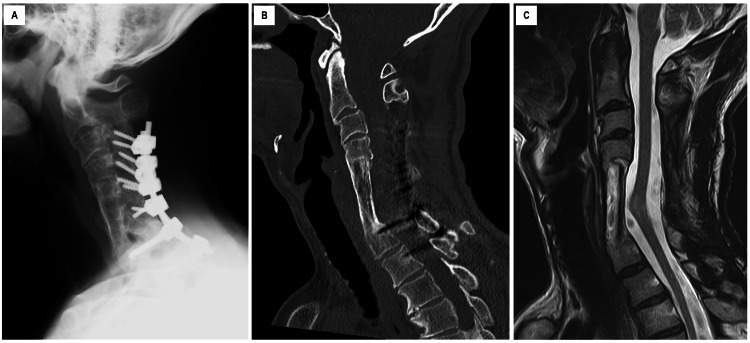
Cervical radiography, cervical plain CT scan, and plain T2-weighted MRI after two years and seven months of follow-up (A) Lateral view of the cervical radiograph. (B) Sagittal view of the cervical CT scan revealed that vertebral bodies from C4 to Th1 were favorably fused with a long autologous bone graft in a good cervical alignment. (C) Sagittal view of cervical T2-weighted MRI revealed sufficient decompression of the spinal cord.
